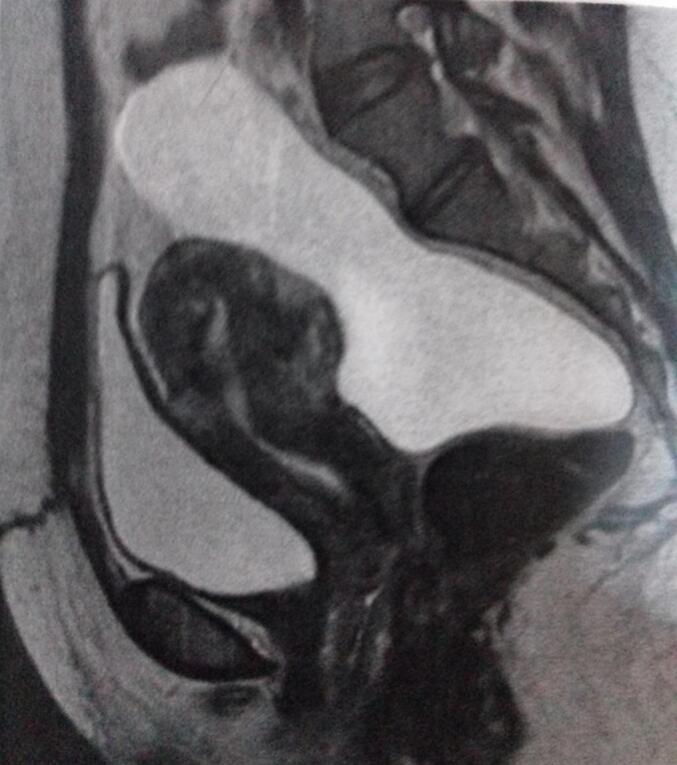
MRI demonstrating cystic lesion with multiple thin internal septa.
